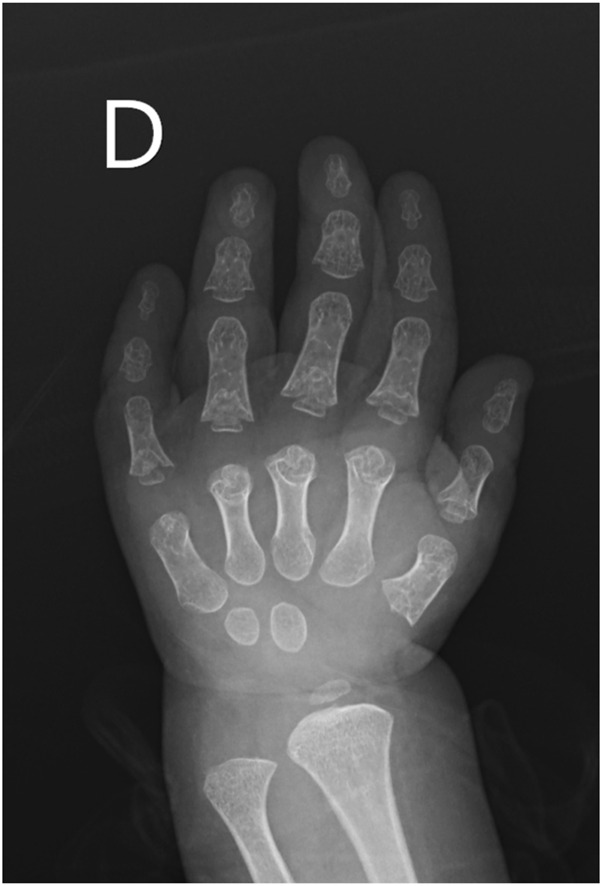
X-ray image of the right hand from the pediatric patient suspected of having Mainzer-Saldino Syndrome (MSS). The image reveals characteristic skeletal abnormalities associated with MSS (cone-shaped epiphyses).
